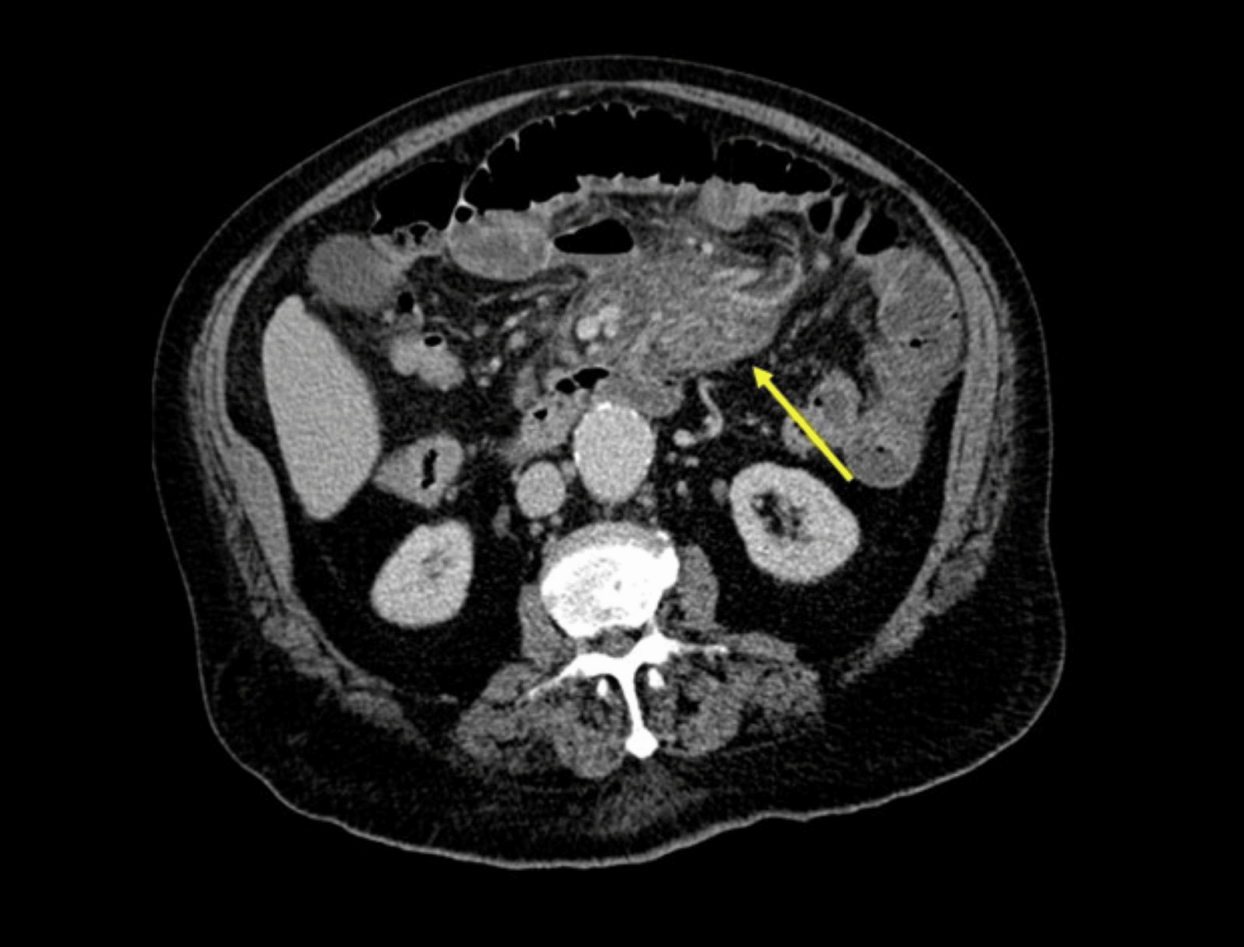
Contrast-enhanced abdominal CT scan in the portal phase showing increased density of mesenteric fat with a pseudomass appearance, engulfing the mesenteric vessels and extending to the adjacent intestinal loops (yellow arrow).
